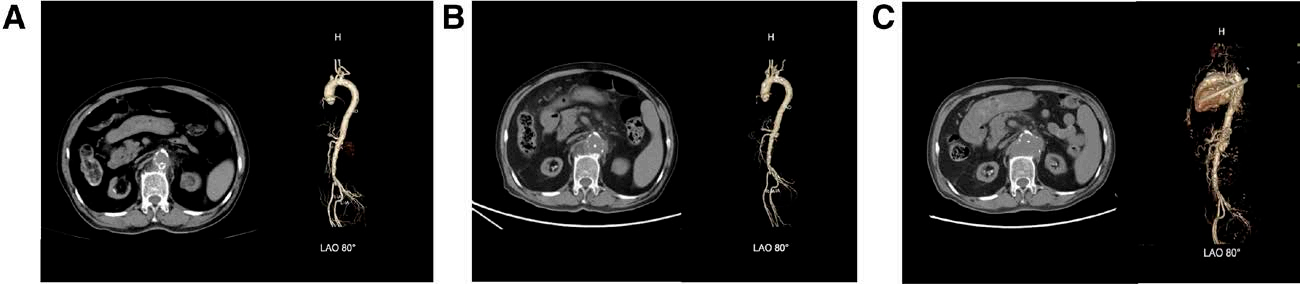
Serial CTA images showing aortic ulcer progression (A. 2024-10-10 CTA, local aortic ulceration with periaortic stranding; (B) 2024-10-22 repeat CTA, progression to penetrating ulcer expanding from 5 to 11 mm with increased inflammation; (C) 2025-01-02 Repeat CTA, multiple penetrating ulcers in the abdominal aorta with formation of a large surrounding hematoma are considered, with significant progression compared to the previous imaging). CTA = computed tomography angiography.
